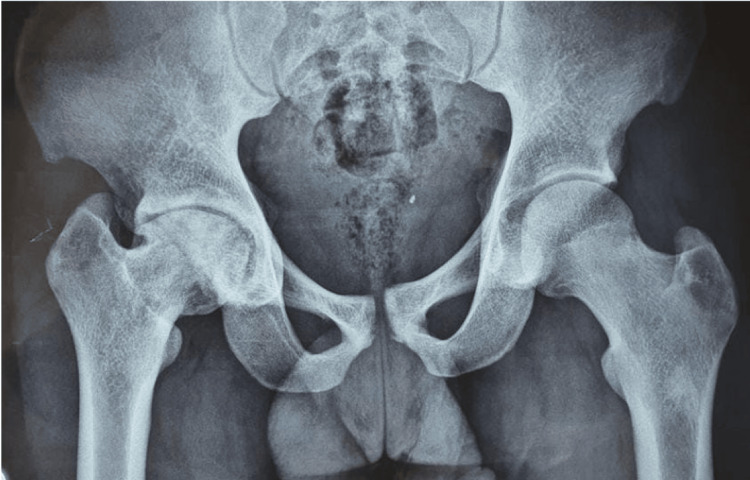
Femoral head osteonecrosis. X-ray showing the loss of sphericity of the femoral head and the loss of joint line regularity associated with subchondral condensation consistent with femoral head osteonecrosis.
